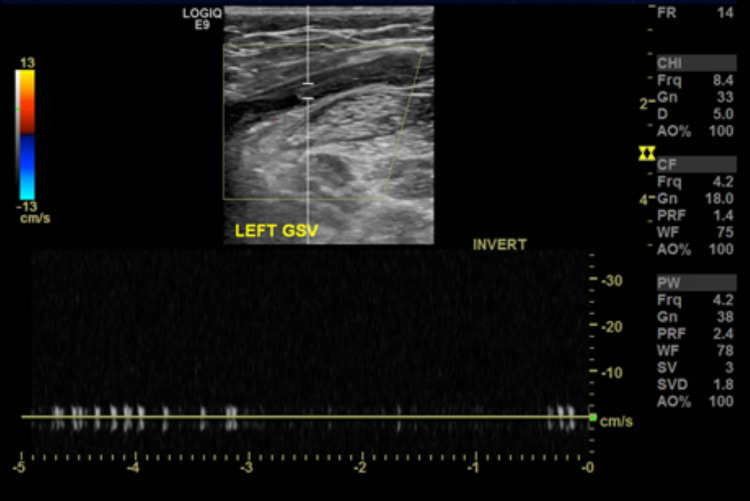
Doppler US from the affected limb. US, ultrasound
